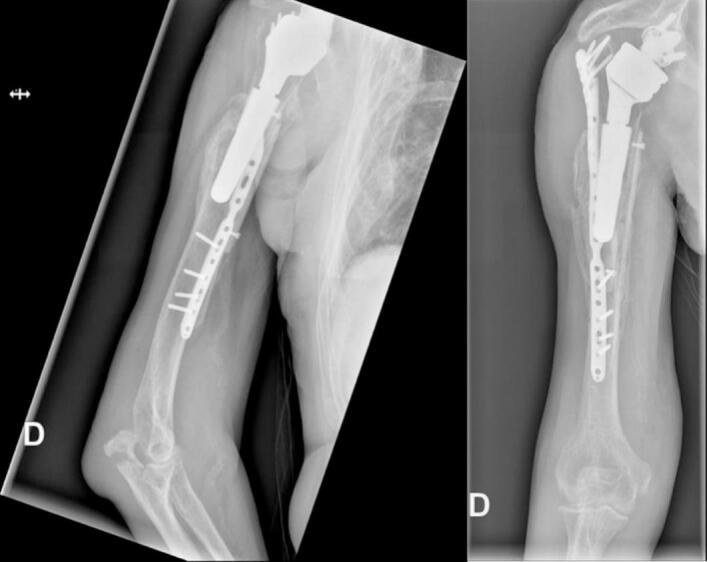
Six months postoperative X-rays after revision ORIF for periprosthetic humeral fracture.
